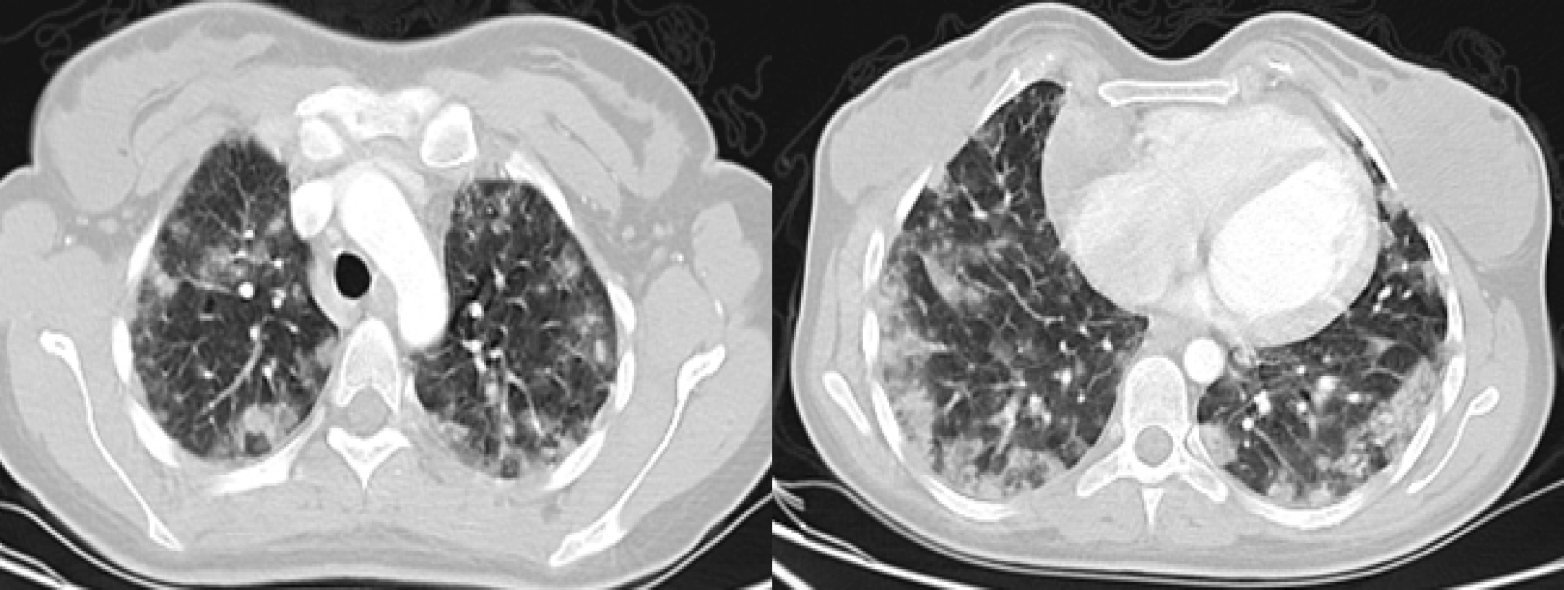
A computed tomography chest scan showed diffuse alveolar ground‐glass infiltrates predominantly in the peripheral lung fields, affecting 60%–70% of the bilateral lung parenchyma.
